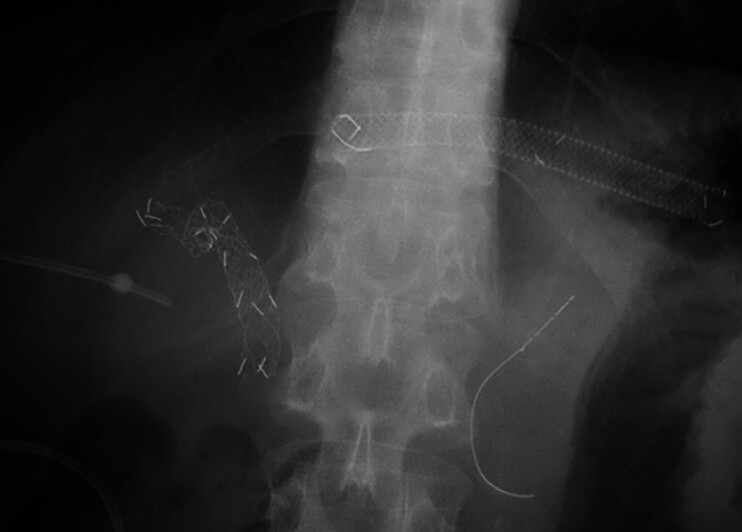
Fluoroscopic image before bridging stenting shows the partially covered self-expandable metallic stent (SEMS) in the endosonographically created route (ESCR) at the B2, two uncovered SEMSs (UCSEMSs) in the anterior and posterior bile ducts, and the percutaneous transhepatic biliary drainage (PTBD) tube in the isolated B6 bile duct.
